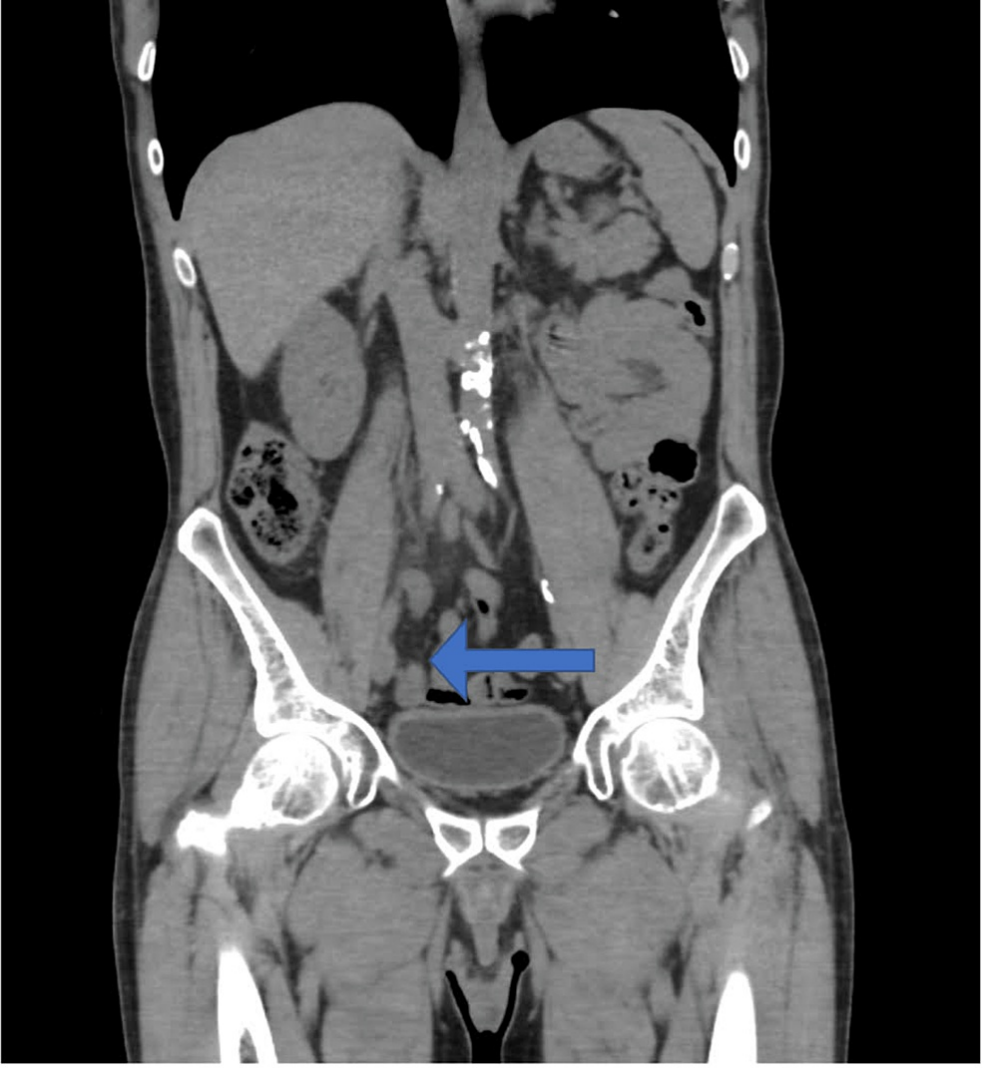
Coronal computed tomography scan done on April 24th.
